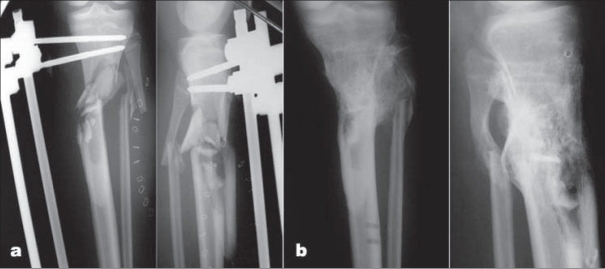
(a) Anteroposterior (AP) and lateral radiographs of (case 19) showing comminuted tibial shaft fracture in an 18 years old male patient. (b) AP and lateral radiographs 6 months after removal of fixator showing radiological union.
